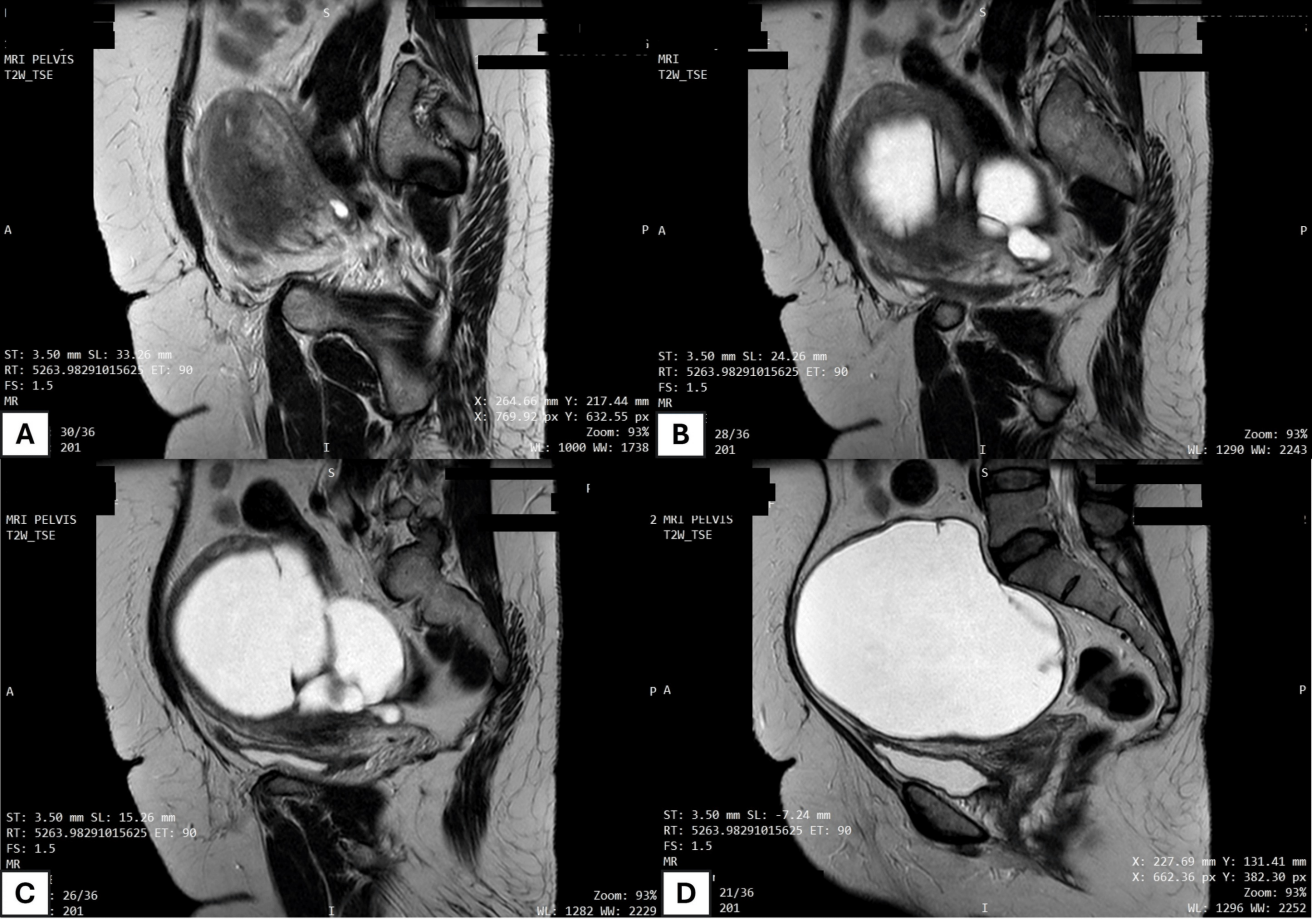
MRI scan showing the sagittal sections of the pelvis (A-D) Lateral to medial sections showing a large cystic lesion in the pelvis, possibly arising from the uterus. MRI: magnetic resonance imaging
